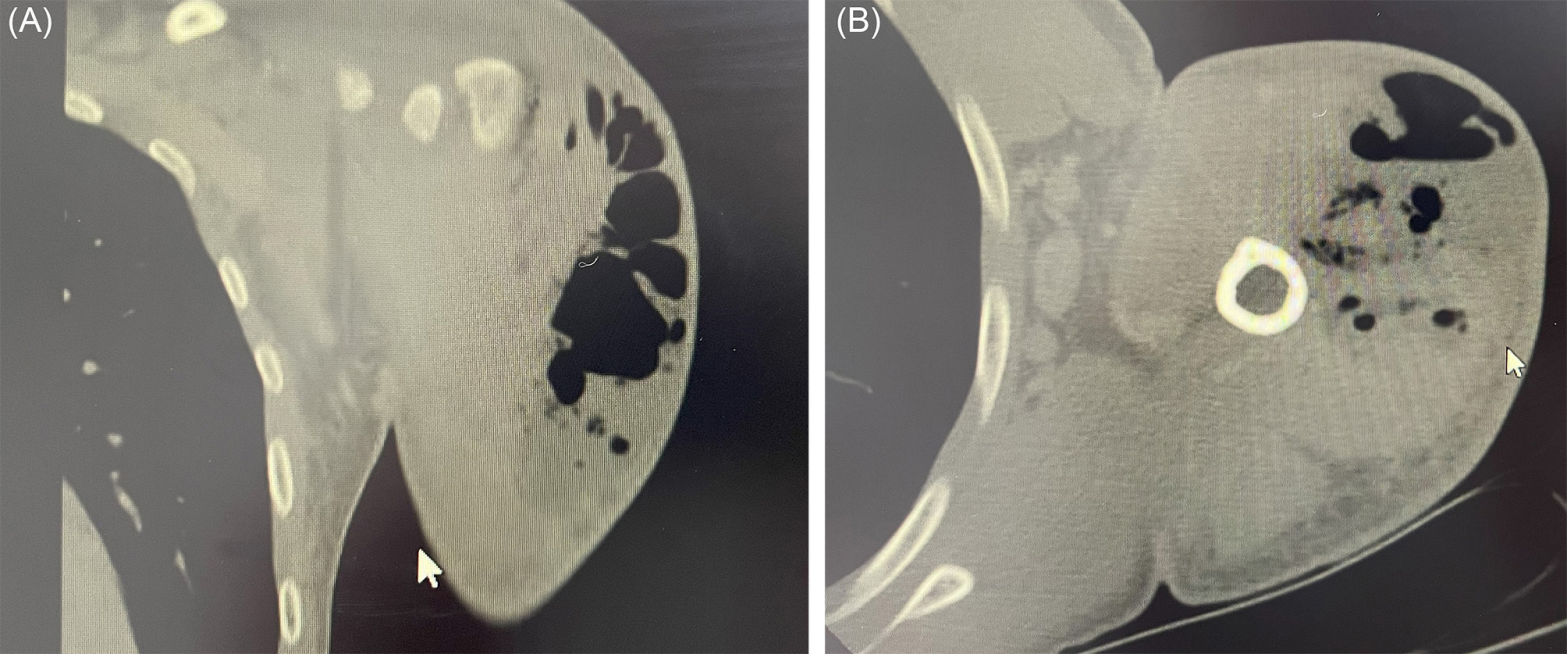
(A) computed coronal tomography: presence of gas. Involvement of rotator cuff tendons, subacromial bursa and acromion; (B) computed tomography with axial analysis: gas in proximal humerus.
